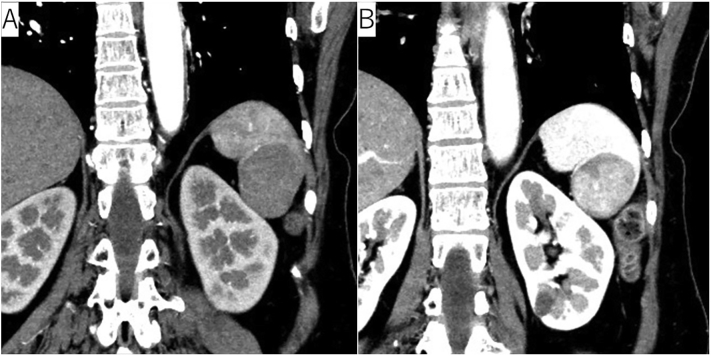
CT (A, arterial phase, coronal; B, portal phase, coronal); enhanced CT showed a tumor in the lower splenic pole with a diameter of 30 mm.
